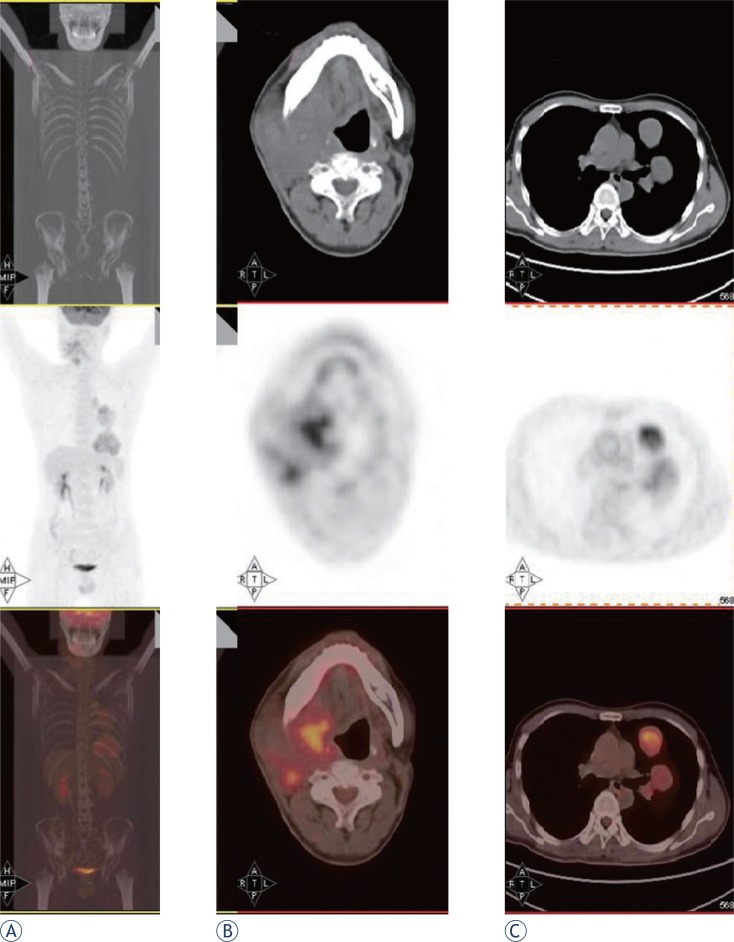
After radiotherapy, repeated chemotherapies and targeted therapies, (a) a whole body scan showed FDG uptake in the right neck and lung. (b) PET/CT revealed a 7.5 × 9-cm mass in the right submaxillary region with high FDG uptake (SUV_max_ = 5.2) involving the right parotid gland, right tongue base, right mandible, jugular vein, carotid artery, and surrounding muscles. (c) Multiple nodules in both lungs, were evident with the largest being up to 6.68 cm in diameter (SUV_max_= 6.02).
